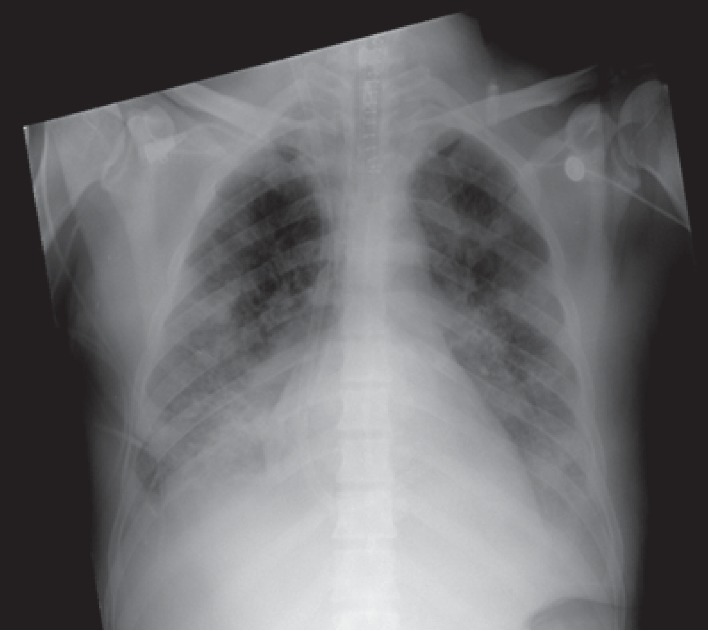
Chest roentgenogram following V-V ECMO institution with right jugular venous cannulation.
